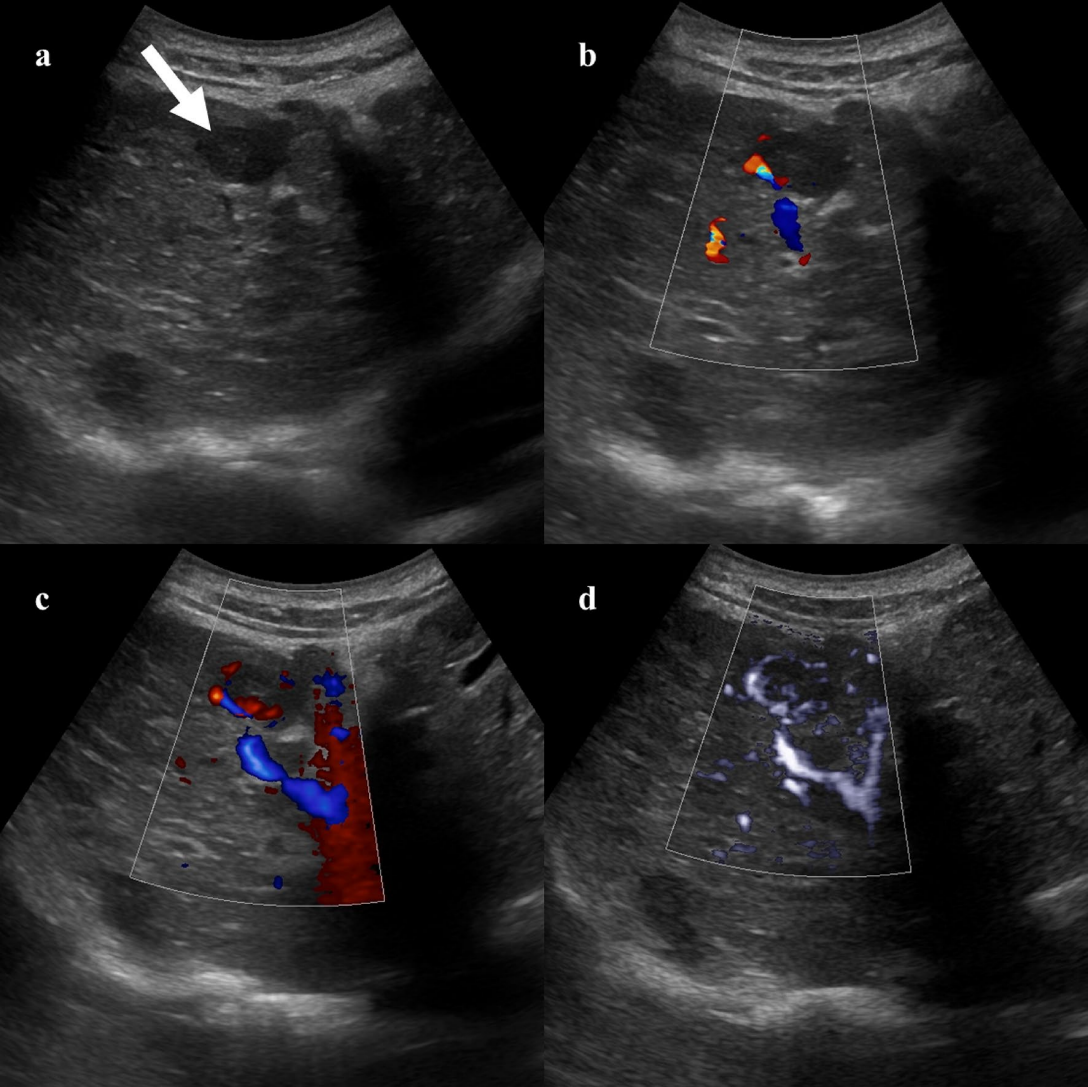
78-year-old man with hepatocellular carcinoma. B-mode ultrasound (a) shows a 2.0 cm hypoechoic lesion in the right hepatic lobe (arrow). Color (b) and power Doppler (c) imaging demonstrate mild peripheral vascularization. Microvascular imaging clearly depicts both peripheral and irregular intratumoral vascular pattern (d)
